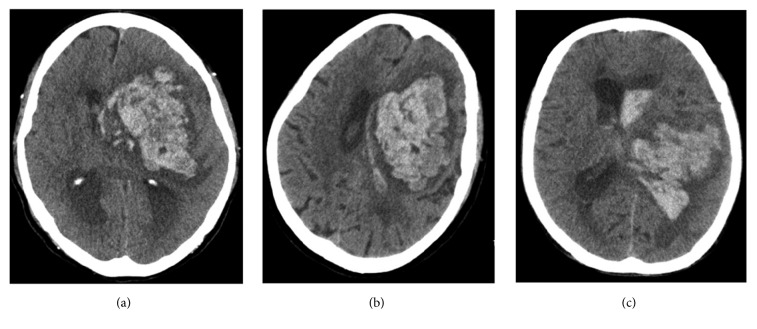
Classic cranial CT scans of Blend Sign II. The whole hematoma was composed of low-density and high-density hematomas without a clear boundary (crossing each other). The difference in the CT measurement value was higher than 10 HU.
